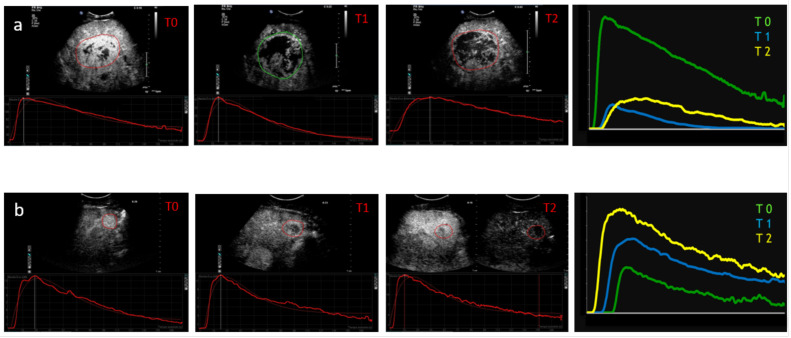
Two examples of HCC patients undergoing systemic therapy. (a) Contrast-enhanced ultrasound with the corresponding time–intensity curve at baseline (T0). At 15 (T1) and 30 days (T2) after the onset of sorafenib therapy, CEUS revealed an increase in tumor necrosis with a drastic reduction in tumor perfusion parameters, as shown by the contrast enhancement pattern and the corresponding time-intensity curve. Time–intensity curves of tumor enhancement are shown at baseline (green curve), on day 15 (blue curve), and on day 30 (yellow curve). It is possible to observe a reduced maximum enhancement and lower area under the enhancement curve early after treatment. Intensity is measured in absolute intensity units (AIU) on the ordinate, while time is measured on the abscissa (in seconds). (b) Contrast-enhanced ultrasound images and the corresponding time intensity curves of HCC lesion before and after treatment with sorafenib are shown in a non-responder patient. The CEUS and the corresponding time–intensity curves of tumor enhancement show no significant difference in perfusion parameters at baseline (T0, green curve), on day 15 (T1, blue curve), or on day 30 (T 2, yellow curve). Intensity is measured in absolute intensity units (AIU) on the ordinate, while time is measured on the abscissa (in seconds).
